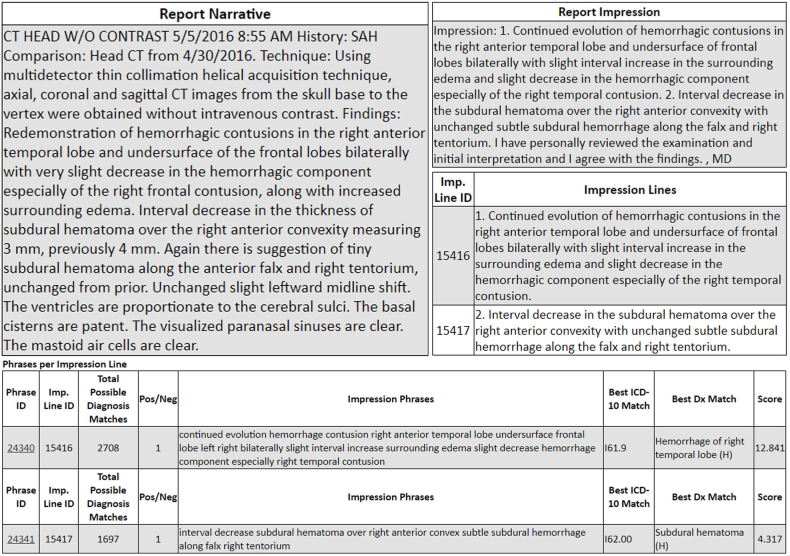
Sample NLP-generated diagnosis codes from a noncontrast CT report. The algorithm separates the Impression of the report (*top right*) into Impression phrases of the report (*middle, right*), which are separated from the rest of the report narrative (*top left*). The generated ICD-10 codes and the ranking/weighting of the likelihood of a “match” are simultaneously provided (*bottom*), with the single best ICD-10 match for that phrase; clicking on the “phrase ID” (*leftmost column, bottom*) provides a list of the other, lower ranked possible matches for that phrase.
